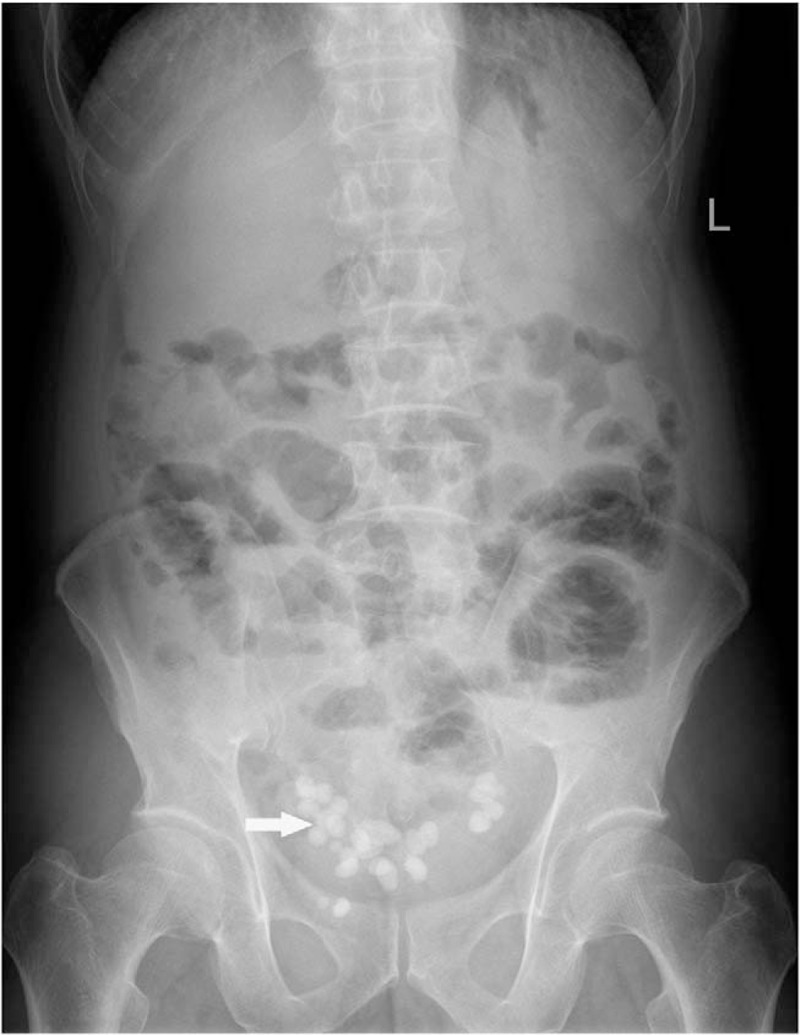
X-ray plain abdominal imaging shows dilatation and air-fluid level within intestine, and multiple high-density nodules with clear boundary in pelvic cavity (arrow).
